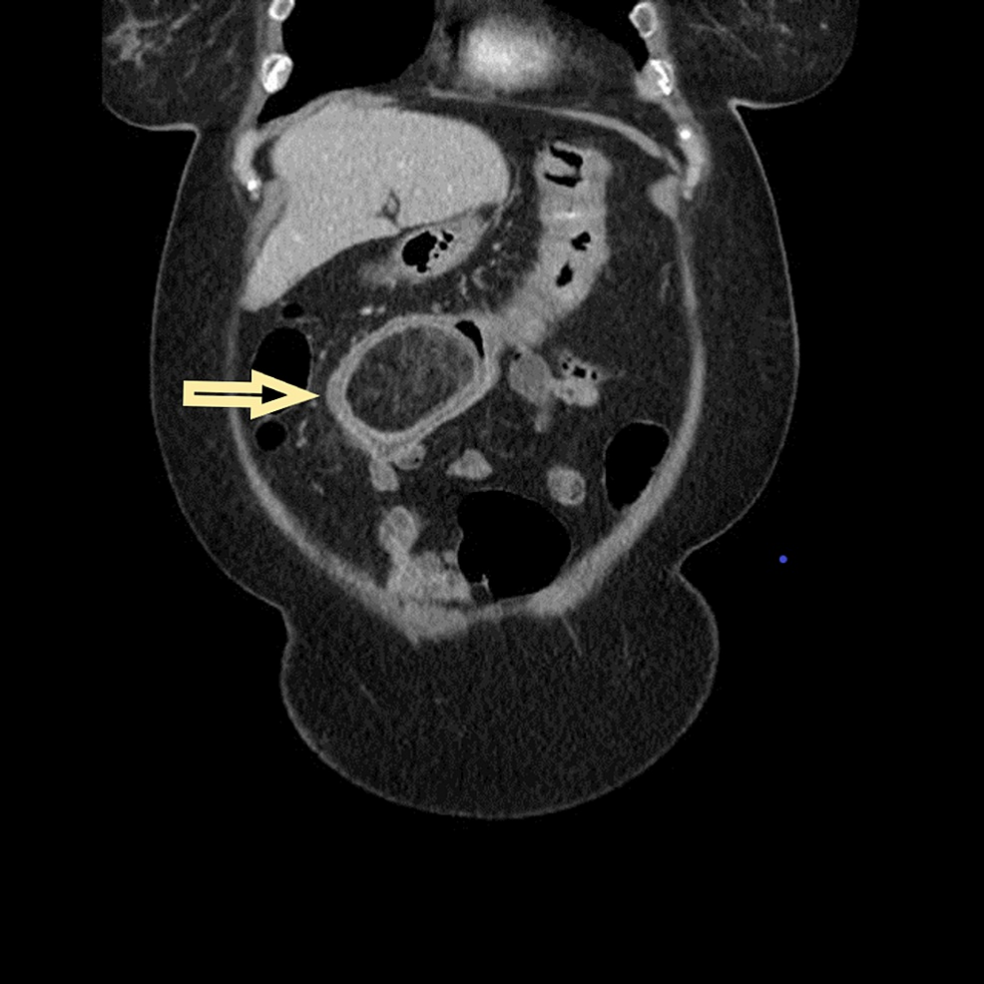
Preoperative computed tomography scan of the abdomen showing a 7.2 x 4.7 x 5.3 cm round mass of fat density representing lipoma within the lumen of the transverse colon. The yellow arrow indicates the giant colonic lipoma in the transverse colon.
